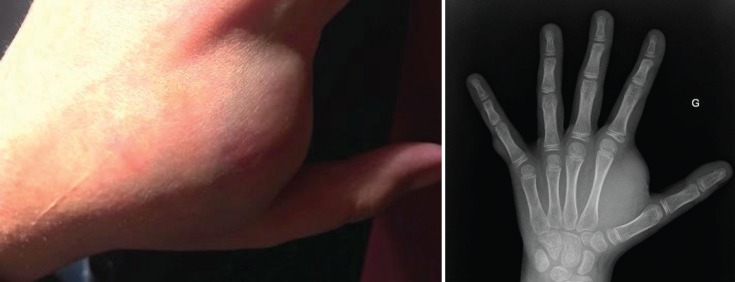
Subcutaneous neurofibroma (left hand): photography and X-ray.
